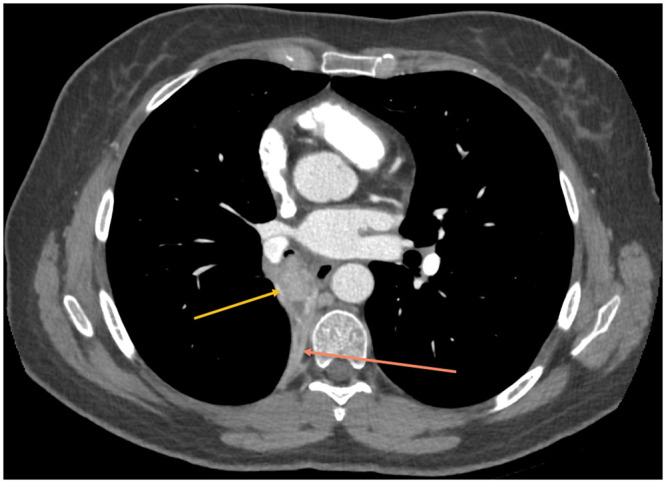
Thoracic computed tomography scan revealing obstruction of the right lower bronchus by a contrast-enhanced intraluminal lesion of 2.5 cm in diameter.
